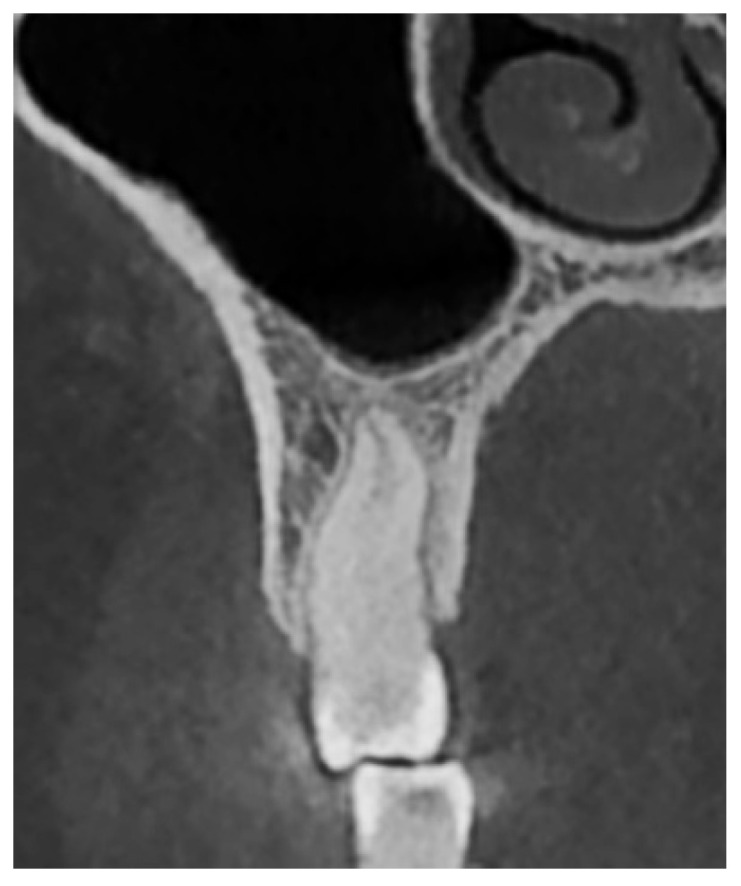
Cross-sectional CBCT along the long axis of tooth 15. Spaced-type relationship between tooth 15 and the maxillary sinus.
